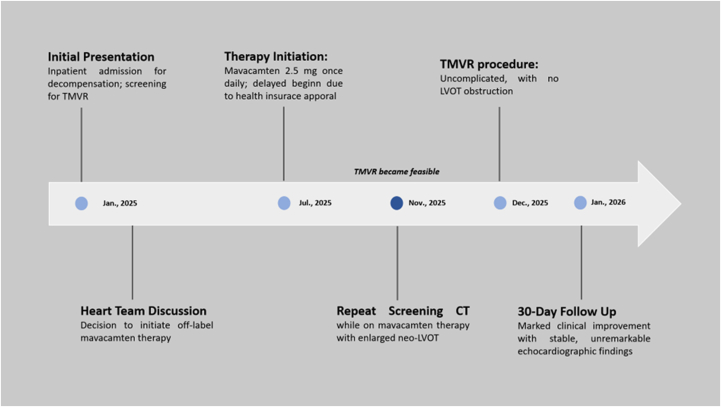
Timeline of the Case CT = computed tomography; LVOT = left ventricular outflow tract; TMVR = transcatheter mitral valve replacement.
